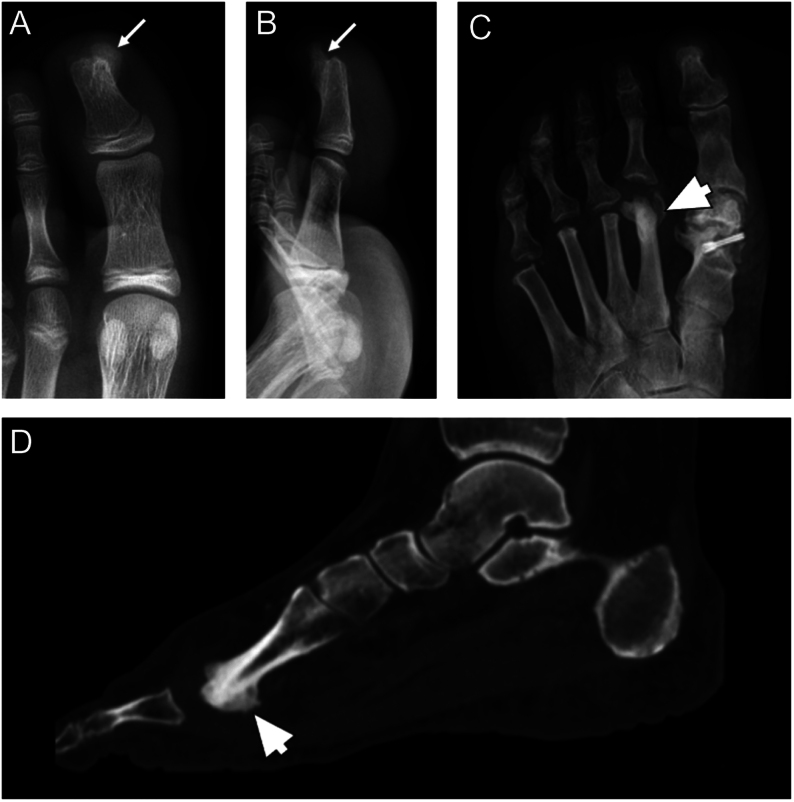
Antero-posterior (A) and lateral (B) radiographs of osteochondroma of the distal foot phalange (white arrows), showing a pedunculated bony mass with continuous growth of medulla and cortex; (C) Nora’s lesion of the second metatarsal bone (arrow’s head) showing mineralizing exophytic outgrowth with a characteristic lack of medullary involvement on antero-posterior radiograph and (D) sagittal CT scan.
